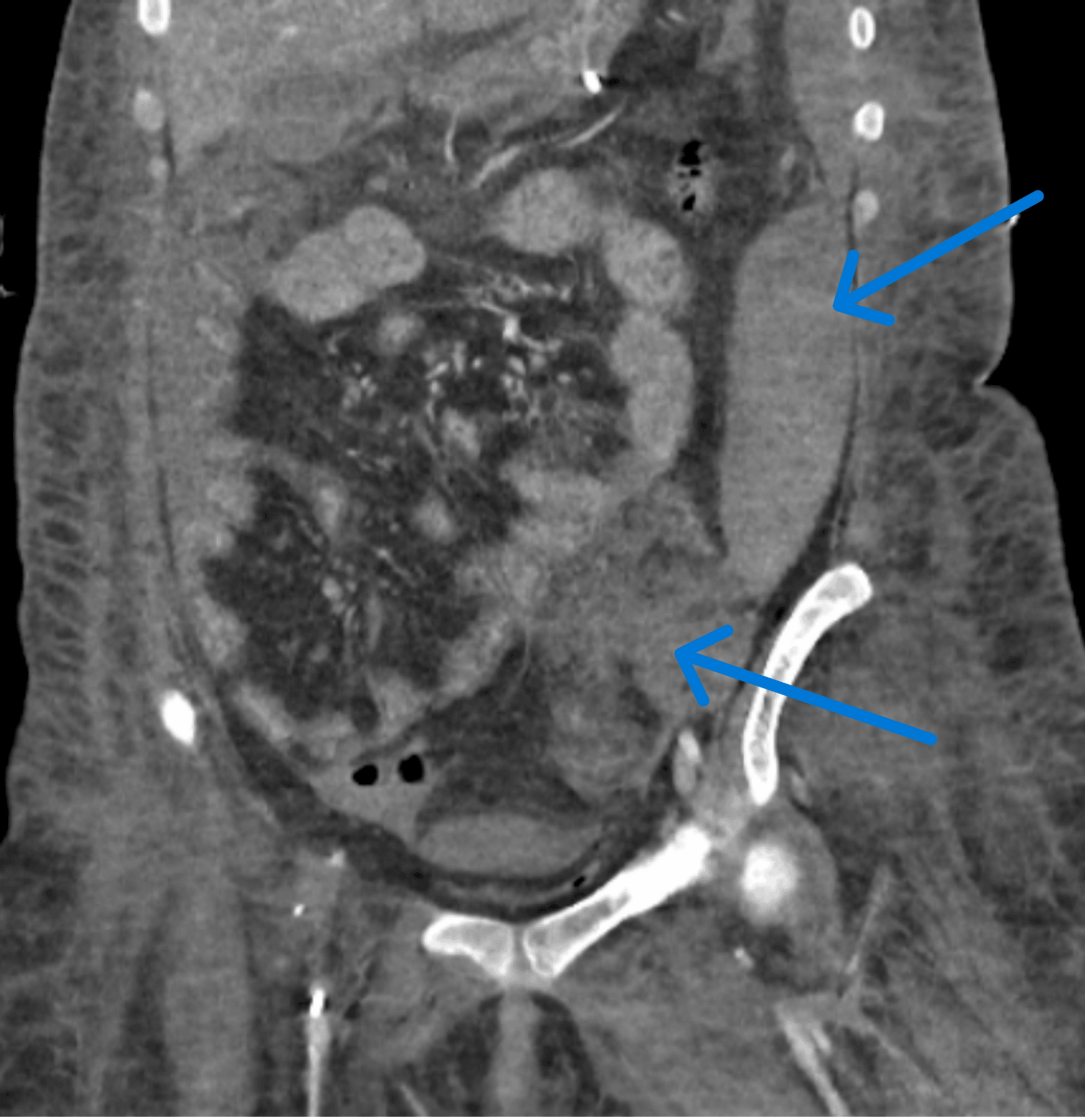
Contrast-enhanced CT of the abdomen and pelvis revealing diffuse swelling of the left iliopsoas muscle showing linear hyperdensities, suggesting an active bleed which is seen extending medially to form a loculated hyperdense collection in presacral region.
